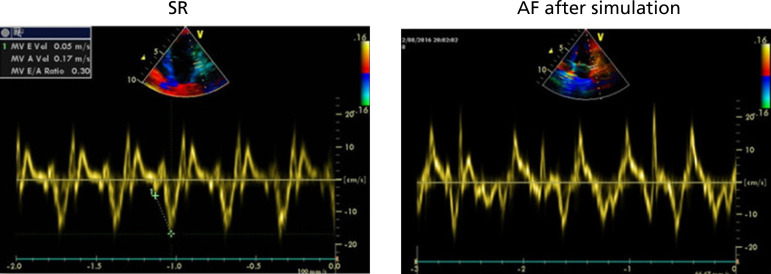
Transthoracic echocardiographies. The left image is the sinus rhythm (SR). The right image is atrial fibrillation (AF) induced by stimulation.
